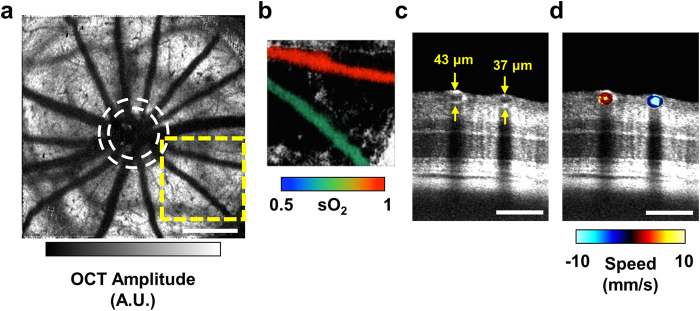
Multi-parameter assessment of oxygen delivery and metabolism with visible-OCT. (a) *En face* maximum amplitude projection of the shadows of the inner retinal vessels. White dashed circles indicate the approximate inner and outer scanning paths for dual-circle Doppler OCT. A.U.: arbitrary units. Scale bar: 500 μm. (b) An artery-vein pair from the yellow dashed box in (a) color-coded according to measured sO_2_. (c) An angular section from the outer circle of the dual-circle scan, showing the artery-vein pair in (b) and their respective measured diameters. Scale bar: 100 μm (d) The same artery-vein pair color-coded according to speed measured by Doppler OCT after phase unwrapping. Scale bar: 100 μm.
